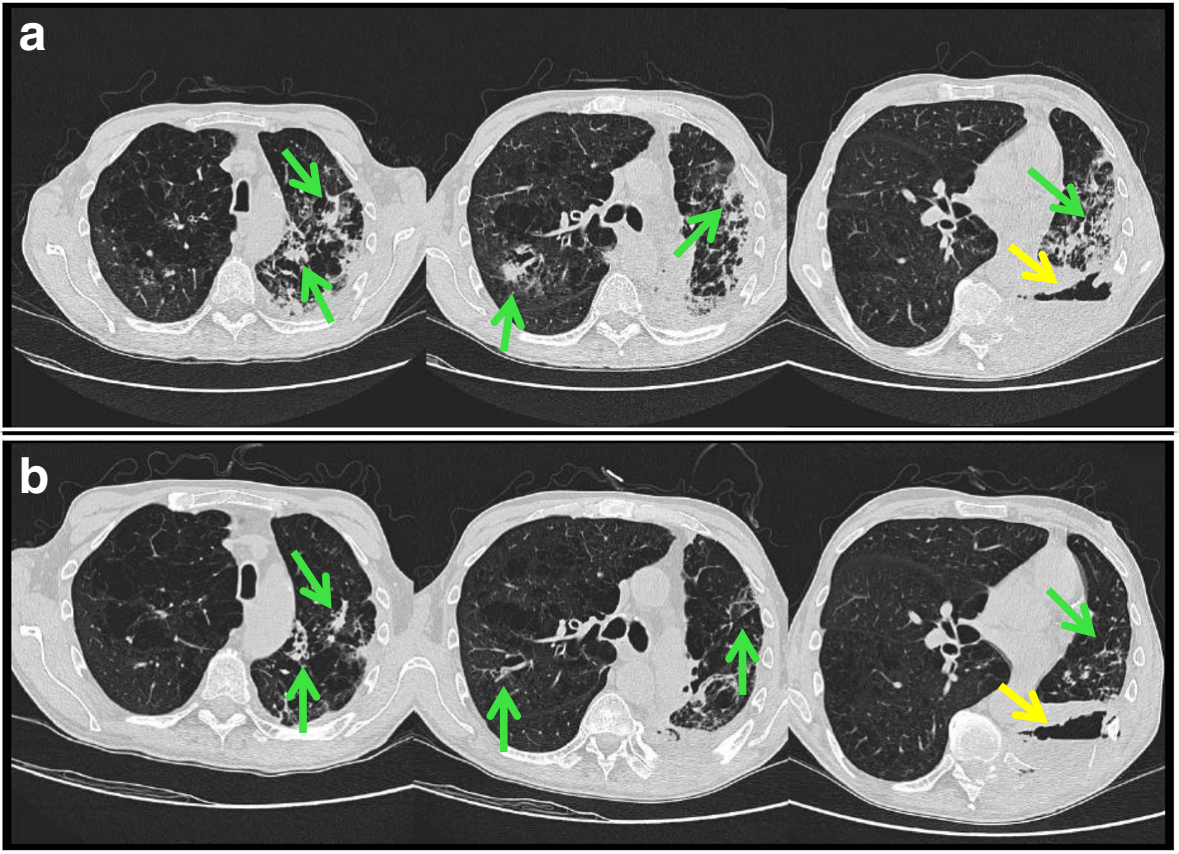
Axial chest CT scans during hospitalization. Pre-treatment images (a) show the cavitary lesion of primary lung cancer (yellow arrow) and multiple bilateral pulmonary consolidations, ground glass opacities and septal thickening prevalent in the left lower lobe relating to inflammatory phenomena (green arrows). Images after 2 weeks of piperacillin/tazobactam therapy (b) reveal a stabilization of the cavitary lesion (yellow arrow) and a reduction in the inflammatory phenomena (green arrows)
